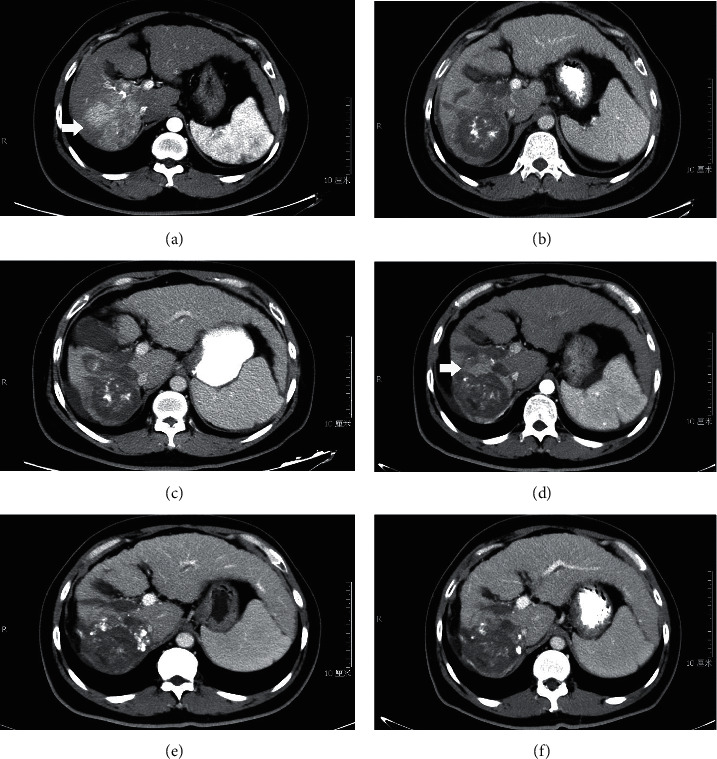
Images of diagnosis and follow-up of a 37-year-old patient with massive HCC and PVTT. (a) CT showing tumor and thromboses in the right branch of portal vein (arrow). (b, c) CT showing no tumor and PVTT enhancement at 1 month and 3 months after first TACE + RFA. (d) CT showing tumor enhancement at 5 months after first TACE + RFA (arrow). (e, f) CT showing no tumor enhancement at 2 months and 5 months after second TACE + RFA.
